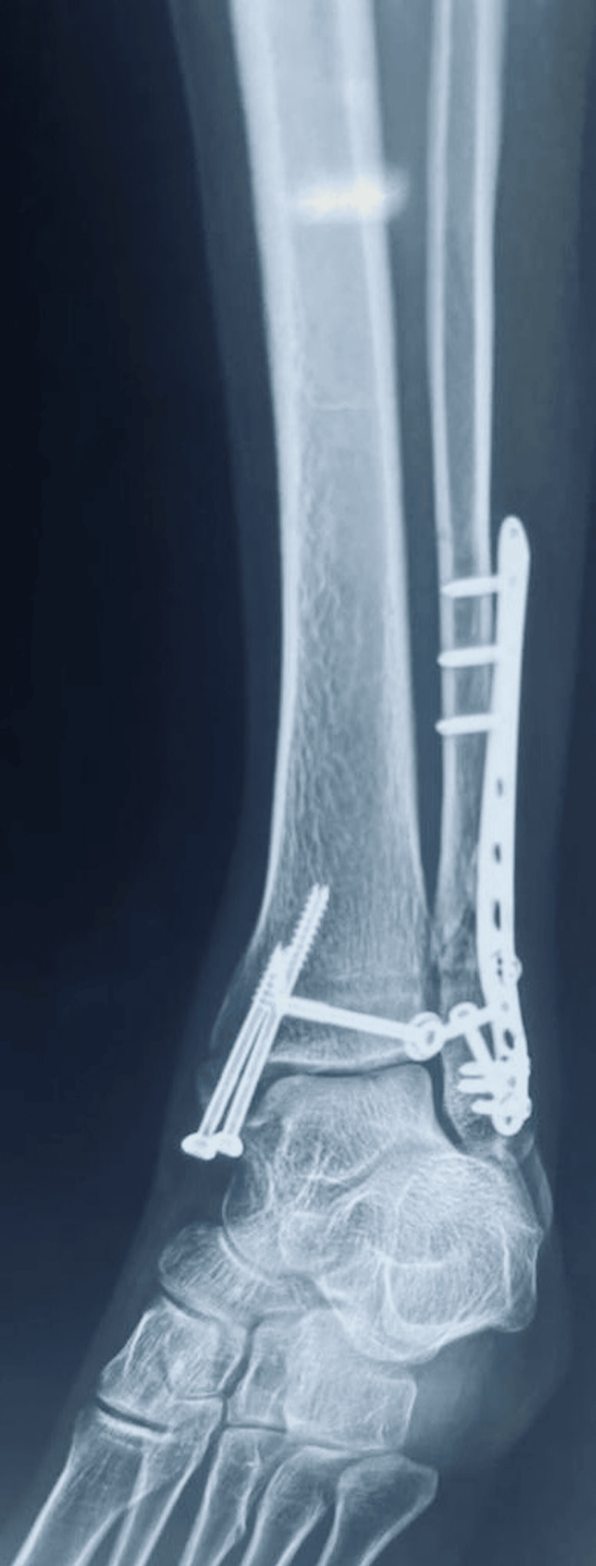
Post-operative X-ray (mortise view) of the left ankle.
